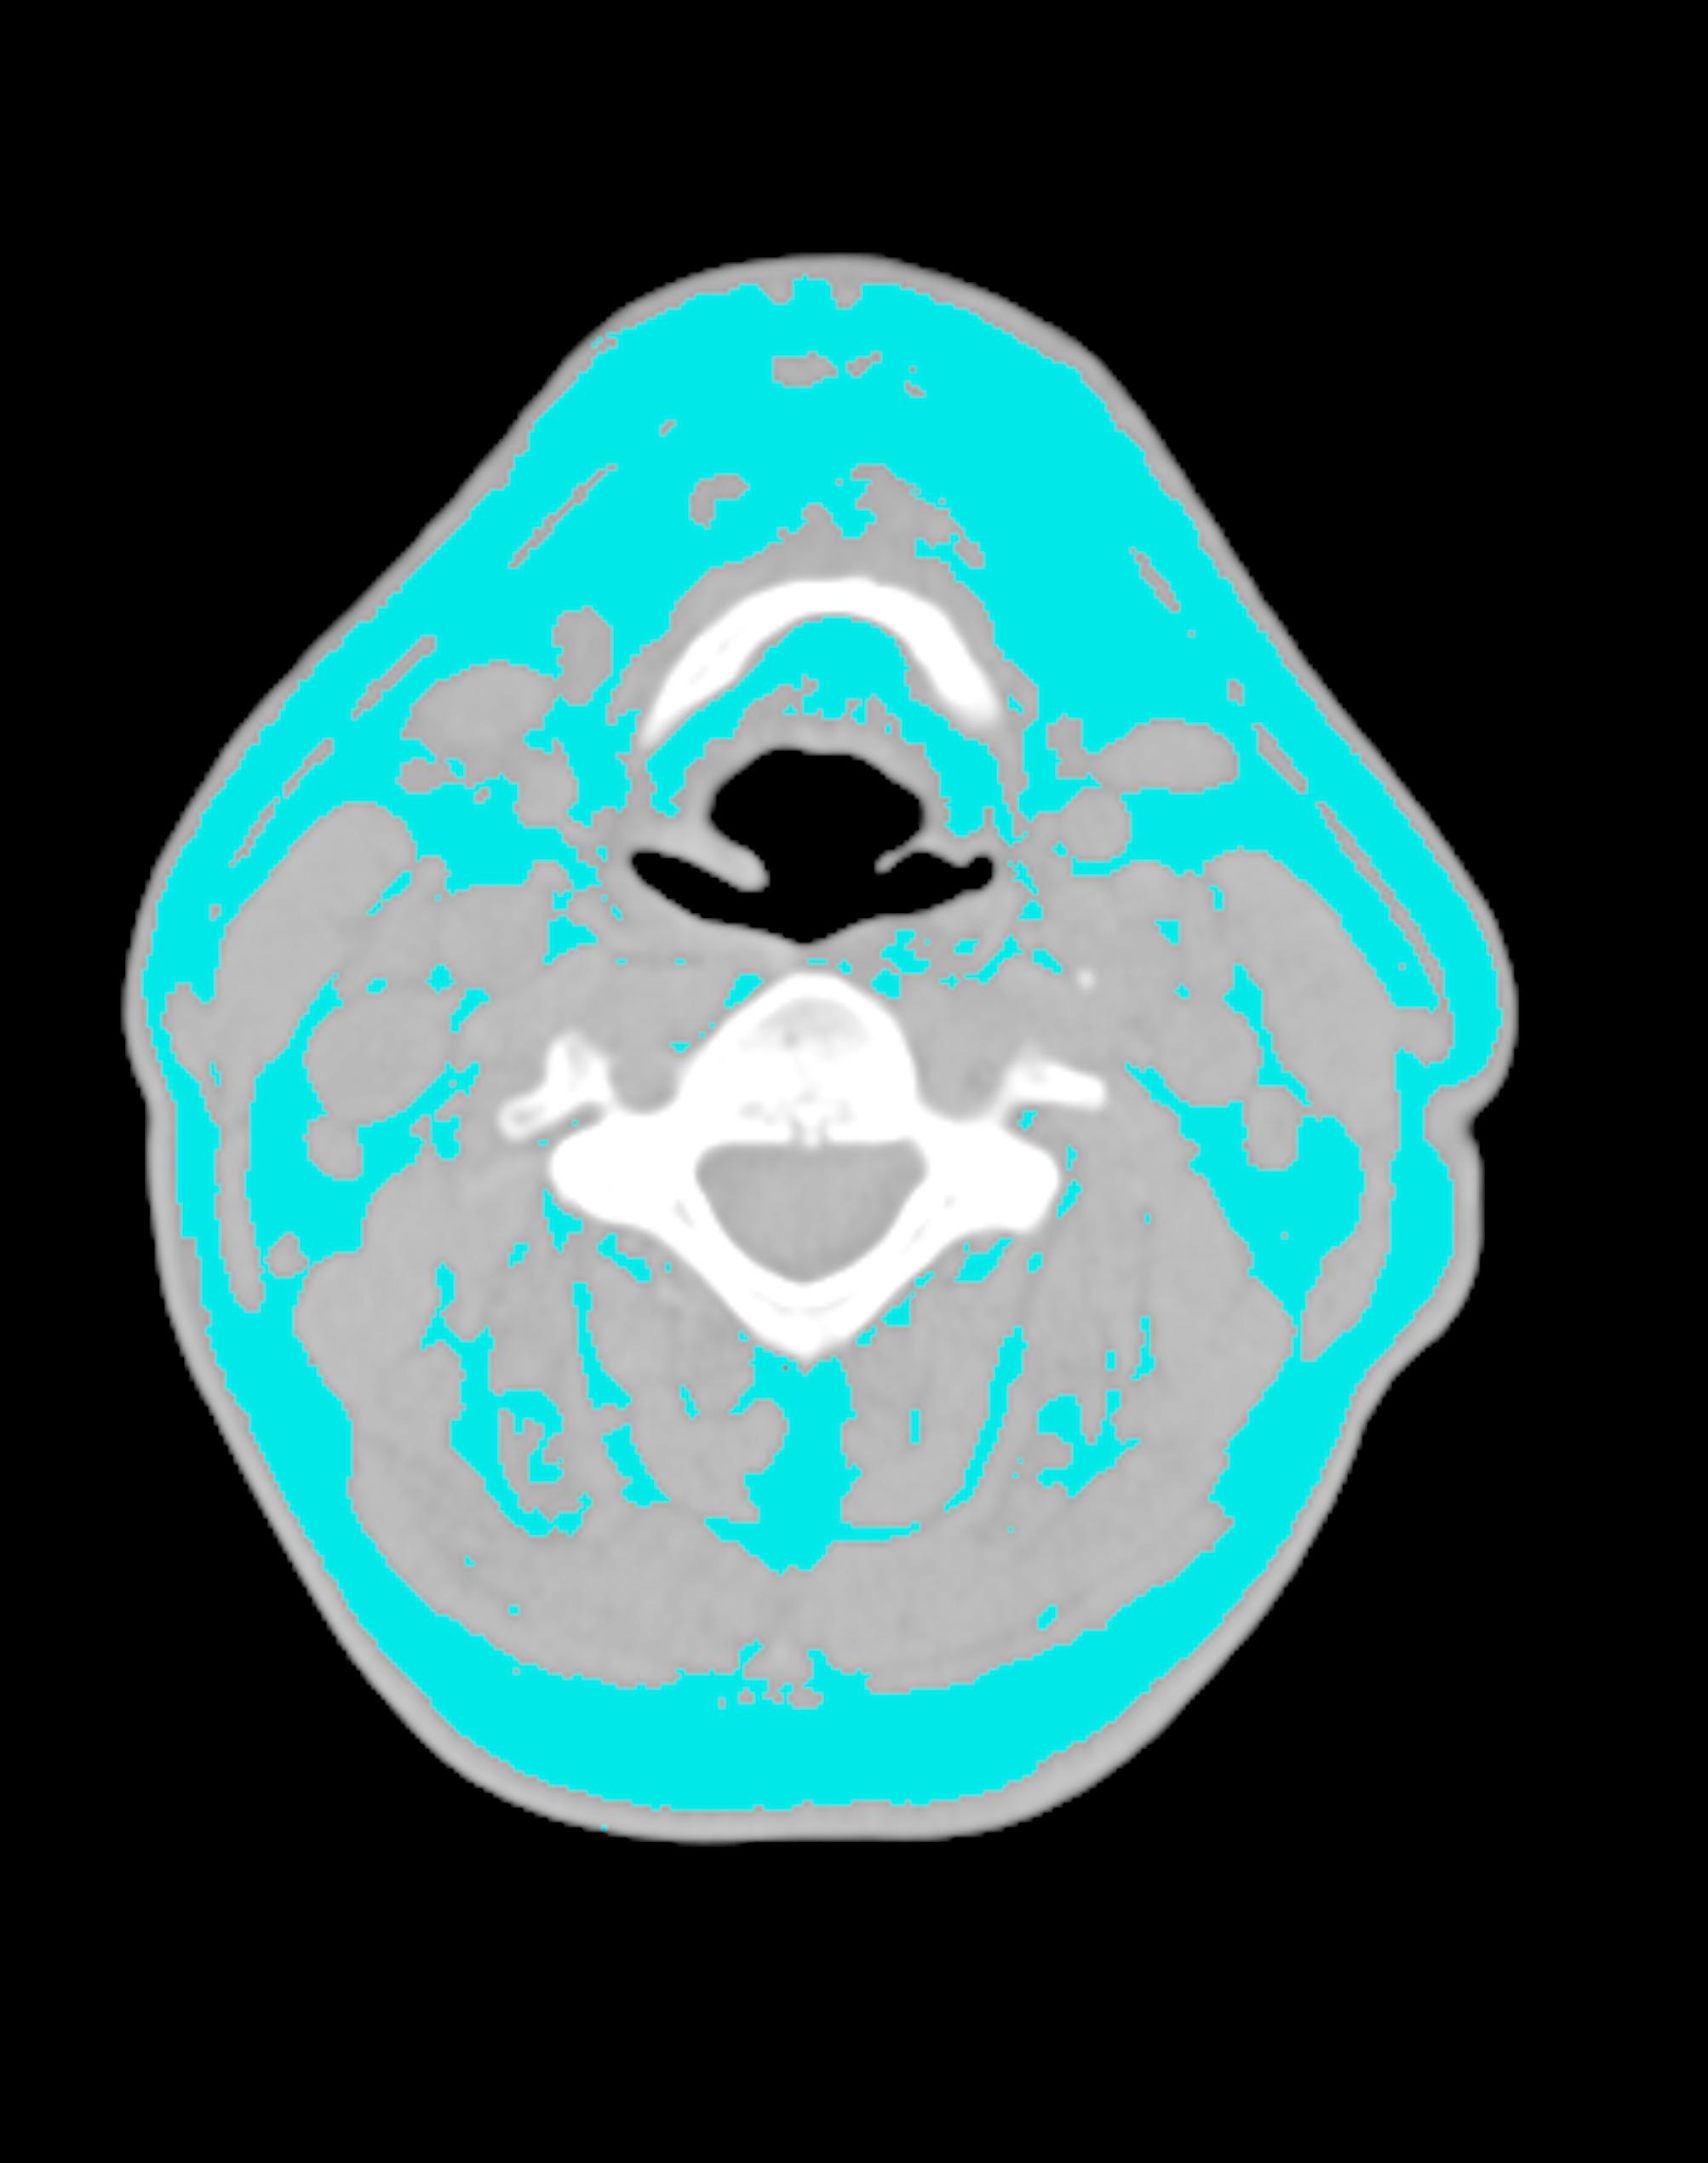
Measurement of NAT Area. Measurement of NAT using Slice-O-Matic V5.0 software (Tomovision, Montreal, Quebec, Canada) with standard thresholds of -190 HU to -30 HU. NAT was assessed by analyzing a single axial neck CT image at the level of the C4 vertebra transverse proces. NAT, neck adipose tissue
